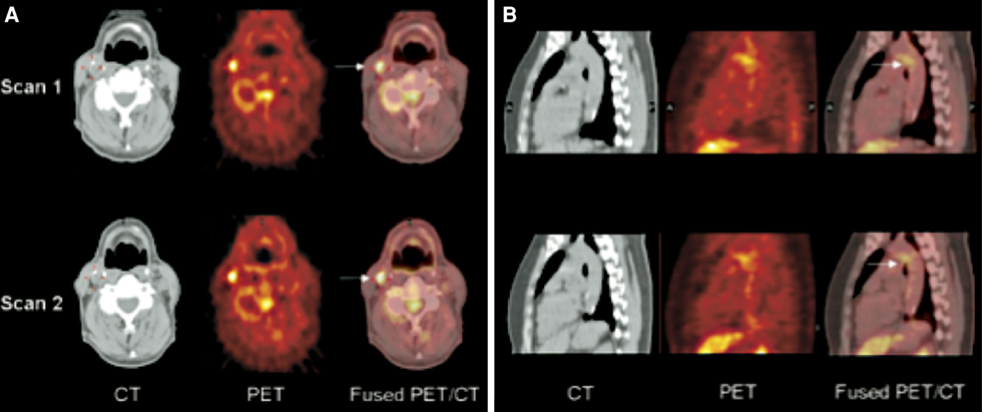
Reproducible carotid and aortic [18F]FDG uptake imaged with hybrid PET/CT over 2 weeks. A reflects carotid CT, PET, and hybrid PET/CT images demonstrating reproducible FDG uptake in the right coronary artery (*arrows*). Similarly, B is indicative of reproducible FDG uptake at the aortic arch and descending aorta of a patient (*arrows*) (Reprinted from Ref^51^with permission from Elsevier)
